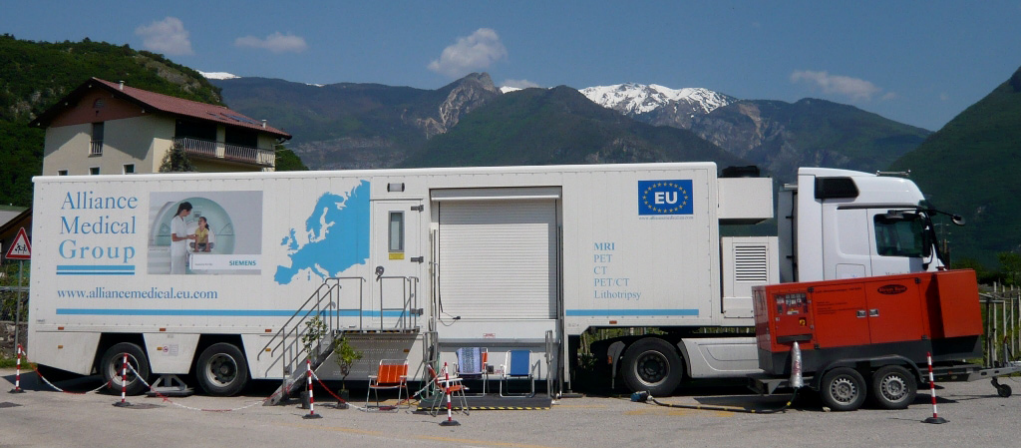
Truck trailer with mobile MRI and external generator in working position.
